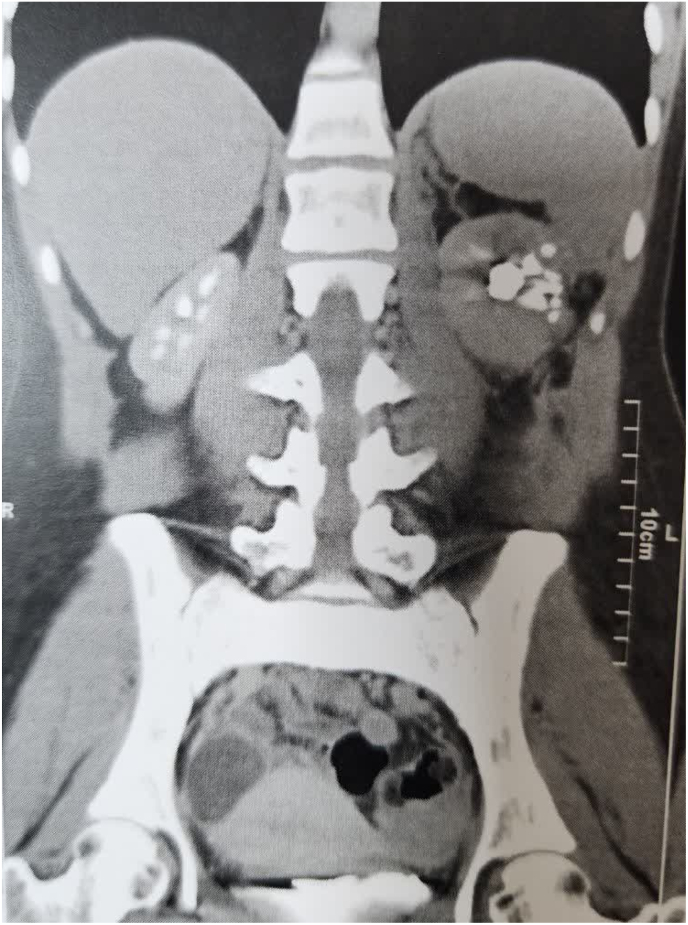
Follow up CT scan with IV contrast revealed normal parenchymal renal tissue and proper function in uro-gram phase without any evidence of local recurrence.
